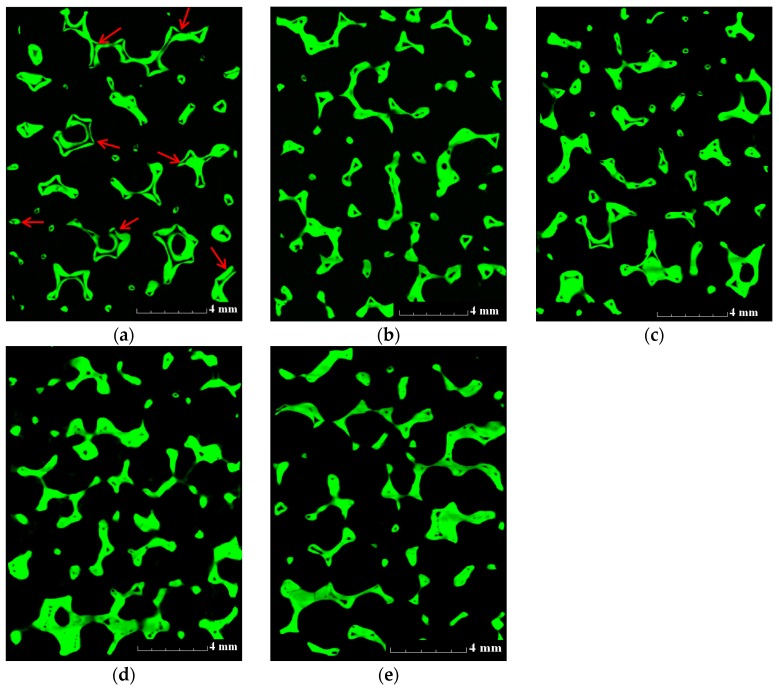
Cross-section images of ZTA foams from µ-CT 3D reconstruction: (a) ZTA20-80 pre-sintered at 1200 °C for 3 h; (b) ZTA20-50-10; (c) ZTA20-50-60; (d) ZTA20-60-10 and (e) ZTA20-60-60.
